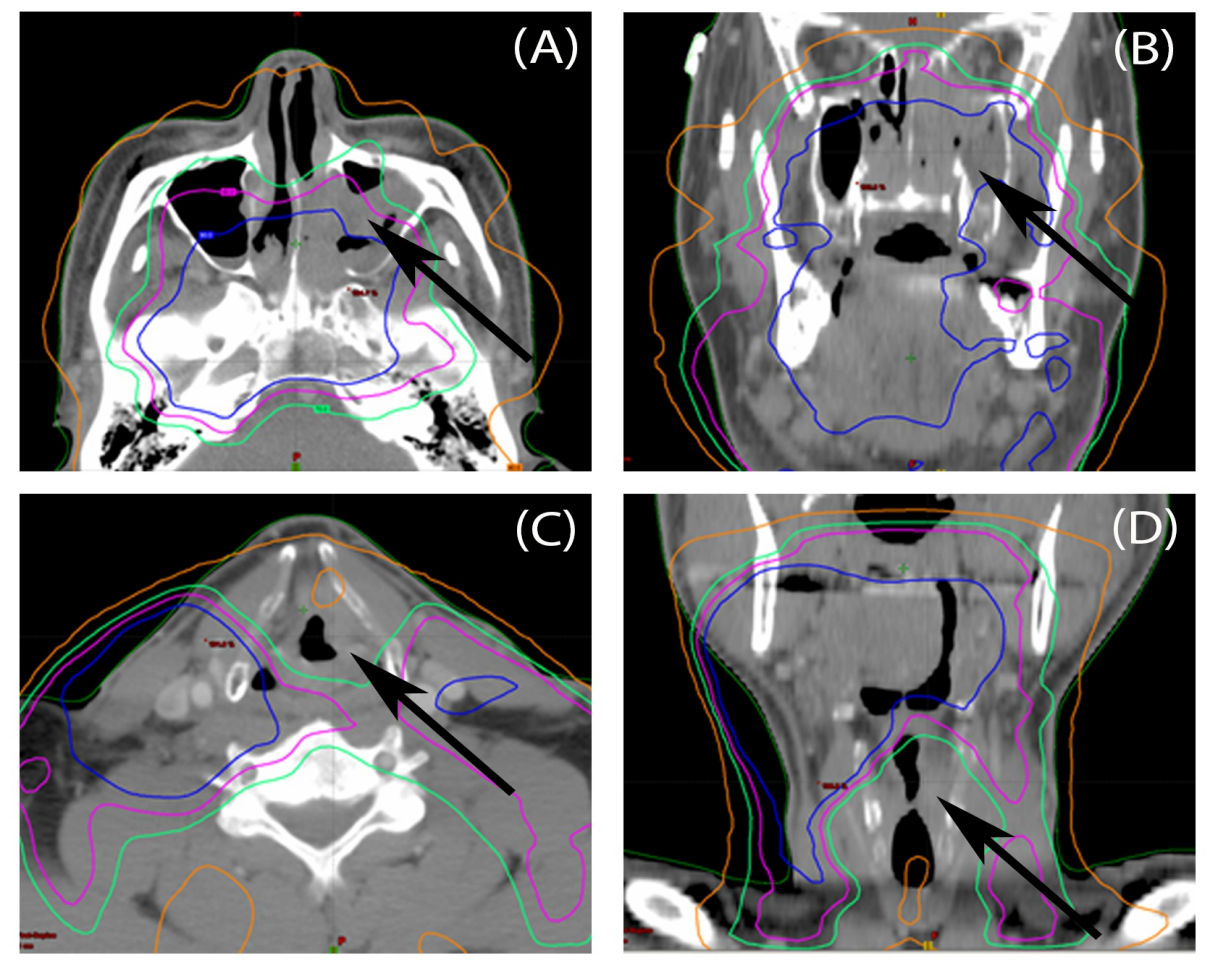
Location of Secondary Malignancy in Relation to Prior Radiation Field for Two Cases CT images of NEC tumors (arrows) in Case 1: Panels A & B, a heterogeneously enhancing polypoid lesion fills the posterior nasal cavity and extends into the maxillary sinus on the left. Case 2: Panels C & D, an enhancing lesion involves the left supraglottic larynx at the level of the false cord, with invasion of the paraglottic space but no destruction of the thyroid cartilage. Isodose lines (blue-90%, purple-80%, green-70% and yellow-40%) from previous treatments are superimposed on diagnostic CT scans, after fusing them in the treatment planning system.
